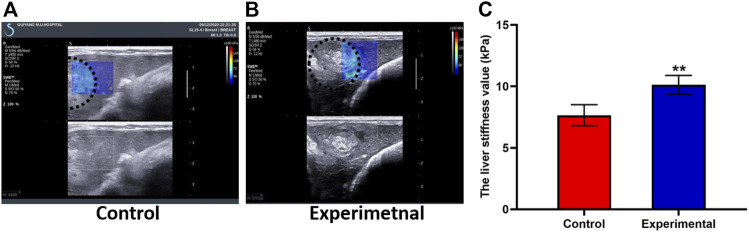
Shear wave elastography (SWE) images from the control group (A) and experimental group (B) demonstrate the ultrasound SWE of liver tissue near the VX2 tumor and of the tumor itself. (C) The liver stiffness value was significantly higher in the experimental group than in the control group. The whole tumor was outlined with a dashed white line. All data are means ± SD. *p <* 0.001.
